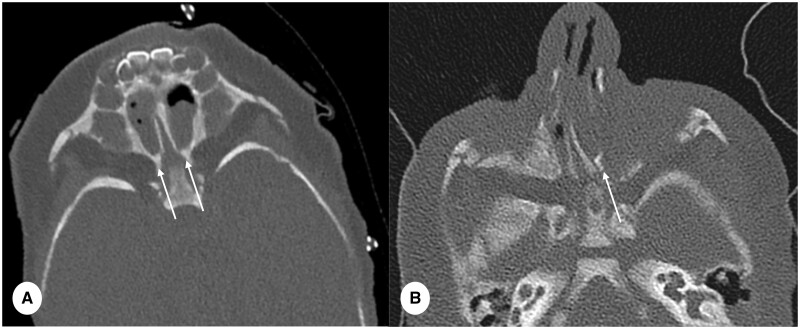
Comparison of CT sinuses for choanal atresia performed with PCD-CT and EID-CT. and a. (A) PCD-CT in a female of 23 days (slice thickness 0.6 mm, reconstruction kernel Hr72 and IR Q3) and (B) EID-CT image in a male of 4.5 months (slice thickness 0.75 mm, reconstruction kernel J70 and IR 3). Image (A) show bilateral bone choanal atresia (thin arrows) and image (B) shows unilateral left choanal atresia (thick arrow). It is important to observe the significantly improved signal-to-noise ratio and enhanced bone edge detection on (A), highlighting the advantages of PCD-CT over EID-CT in the assessment of choanal atresia in paediatric patients.
